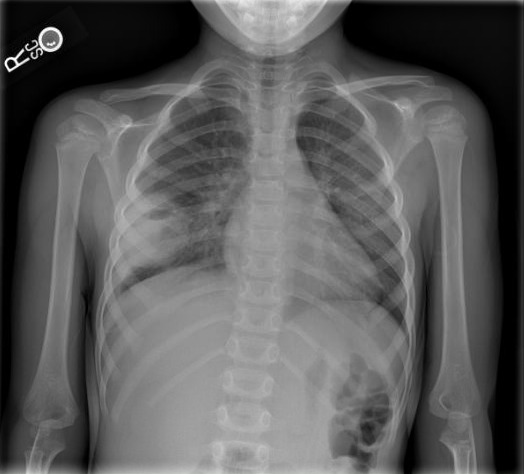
Initial Chest Radiograph.
